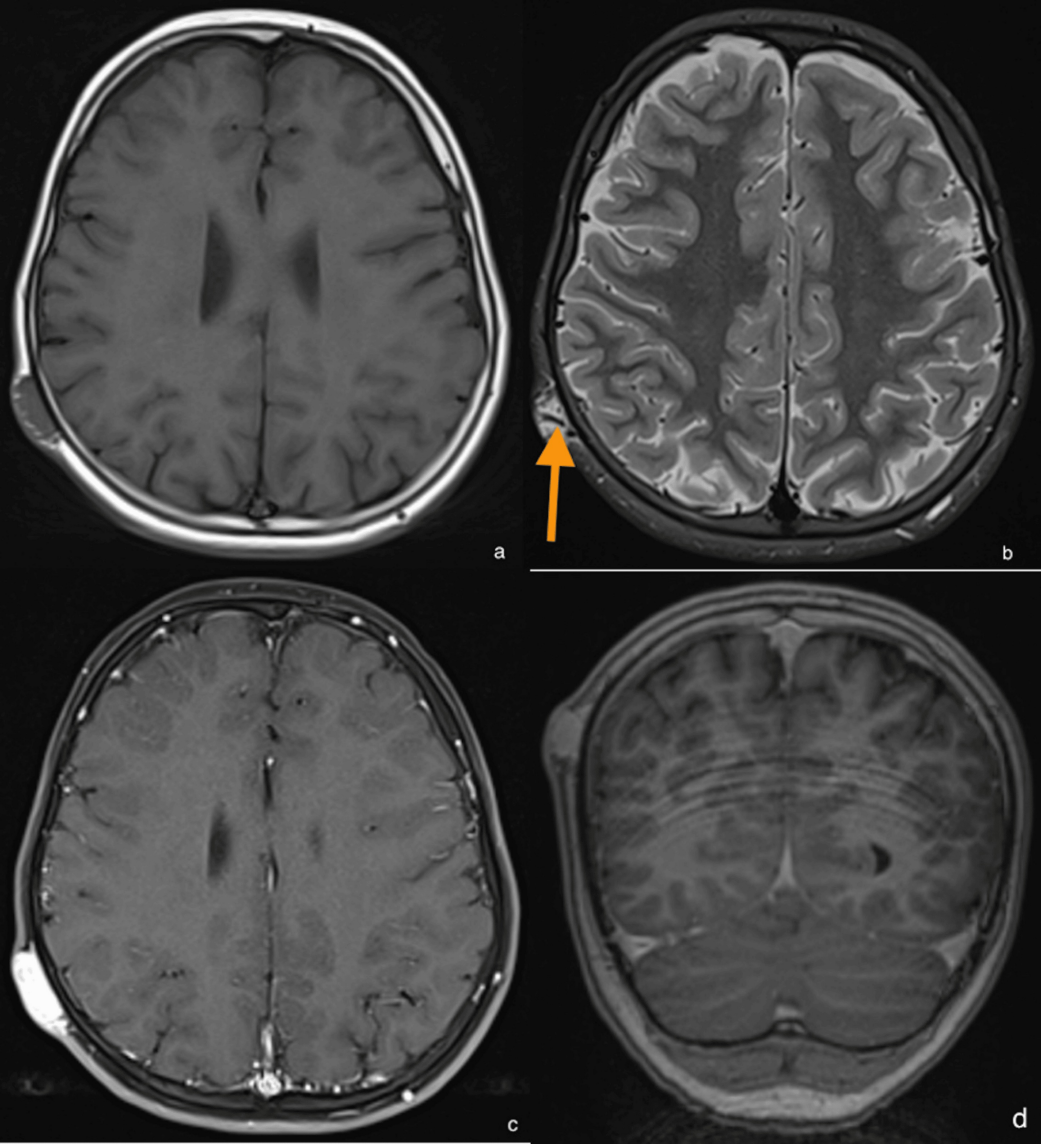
MRI caracteristics (a) Axial T1 hyposignal; (b) axial T2 STIR shows a big intratumoral vessel; (c) and (d) axial and coronal T1 gadolinium hypersignal
